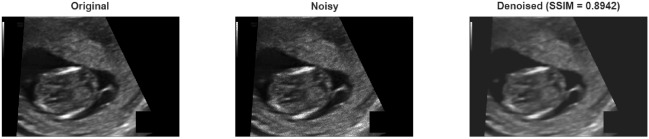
A sample result from fetal ultrasound dataset with Gaussian noise.
